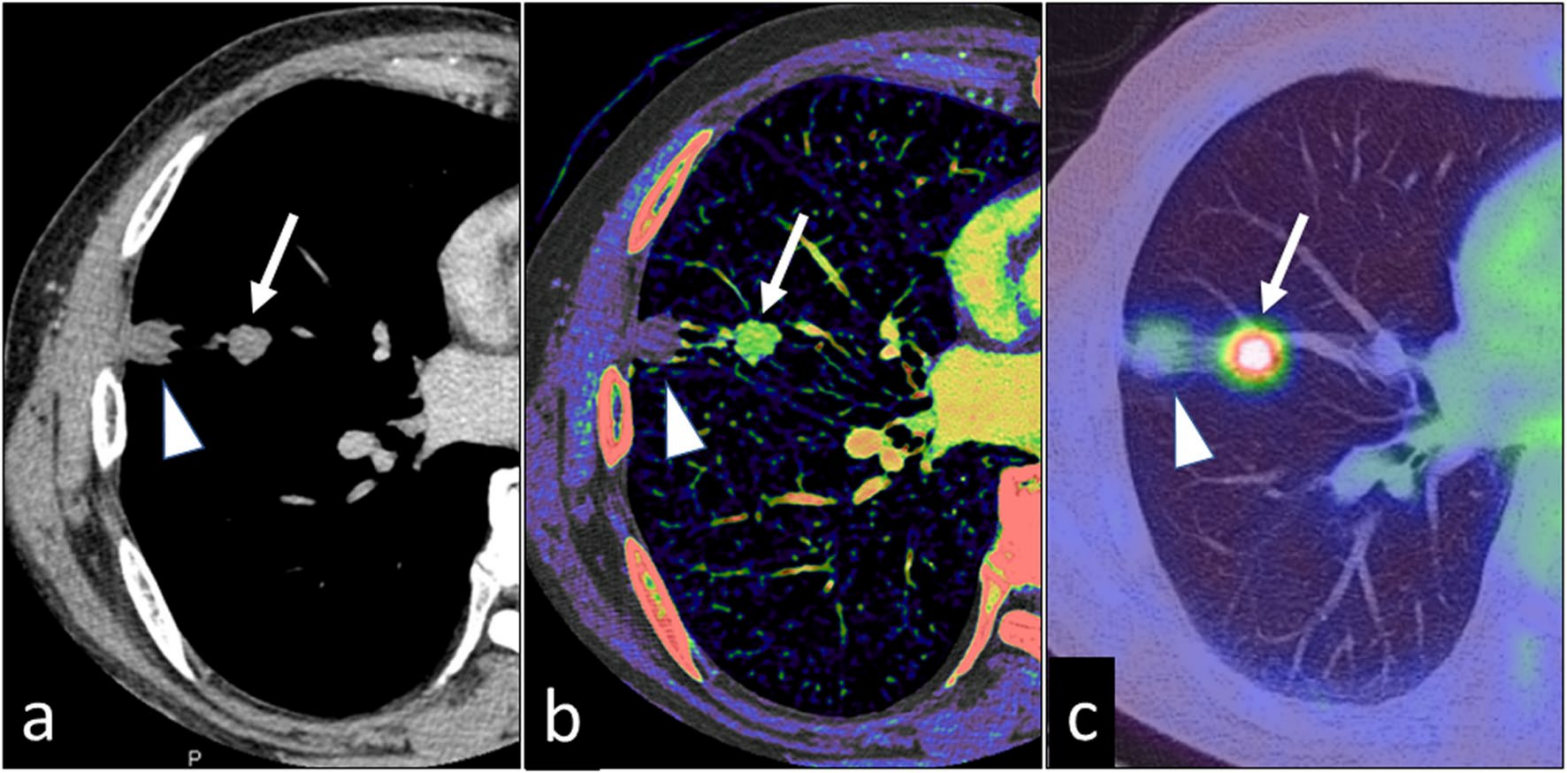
A 76-year-old man with two pulmonary nodules in the right lung. On the 70 keV virtual monochromatic image (a), the degree of enhancement is similar for both nodules. The iodine map (b) shows that the nodule at the proximal site (arrow) is highly vascular; the peripheral nodule (arrowhead) is not enhanced. Pathologically, the proximal nodule was identified as an adenocarcinoma and the peripheral nodule as an infarction. c PET-CT image (the maximum standardized uptake value of the tumor was 6.4)
